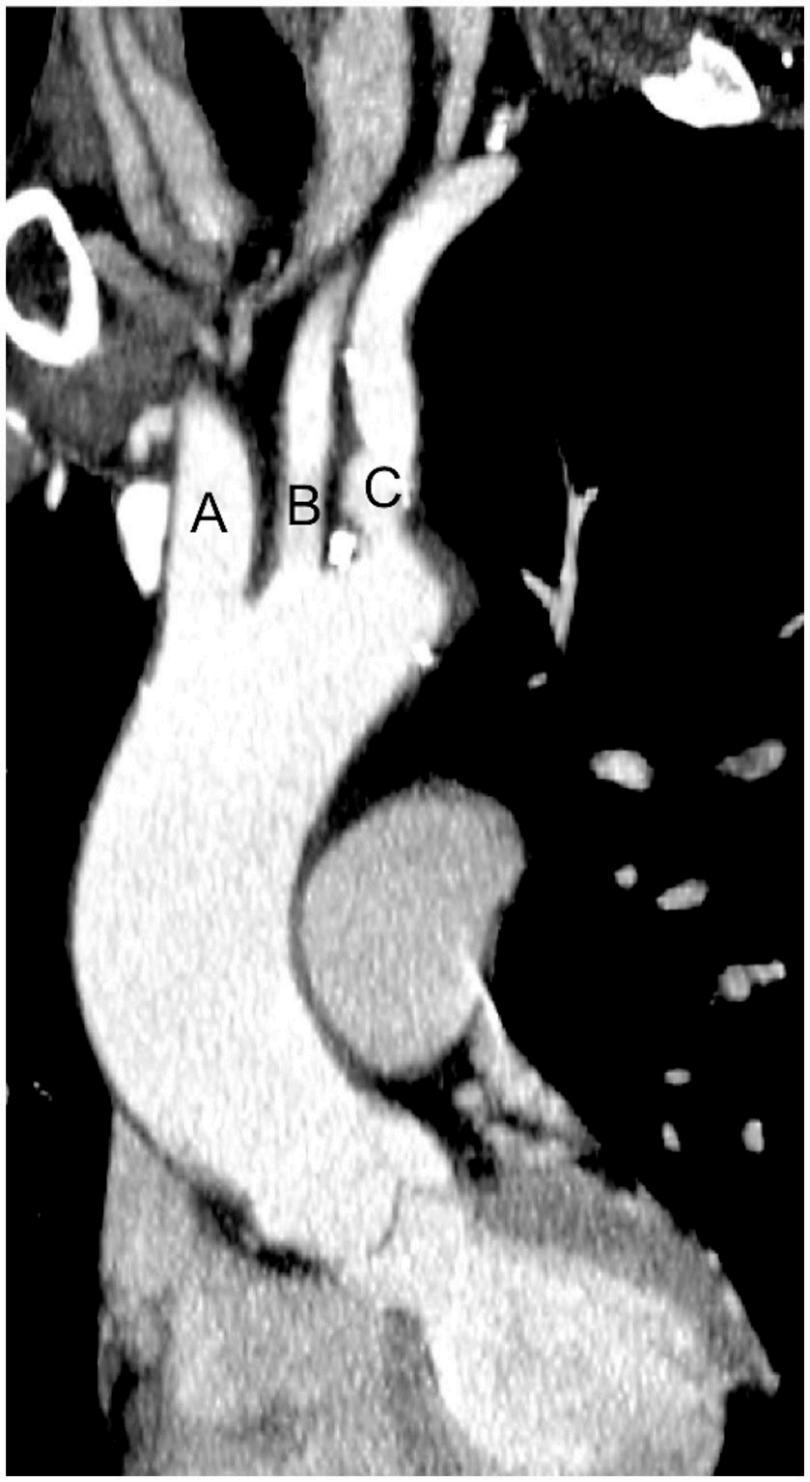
Computed tomography image of ascending aorta and supra-aortic vessels. (A) Brachiocephalic artery; (B) Left common carotid artery; (C) Left subclavian artery.
